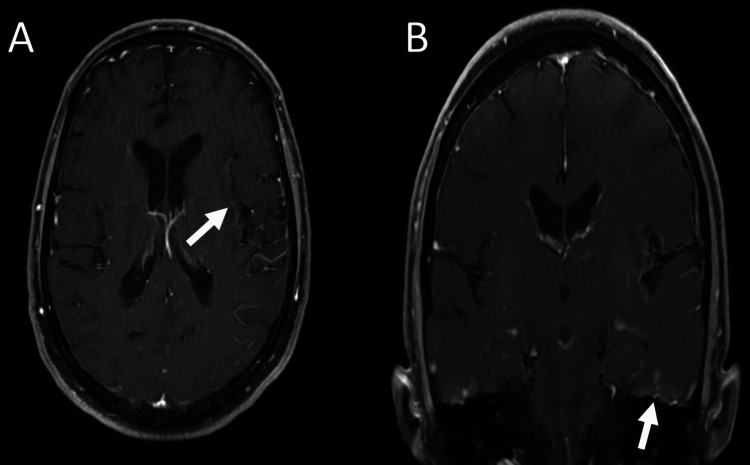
T1 post-gadolinium MRI of the brain. Axial and coronal sequences (A and B) revealed faint enhancement in the left temporal lobe and insular region, with associated leptomeningeal enhancement. MRI: magnetic resonance imaging
